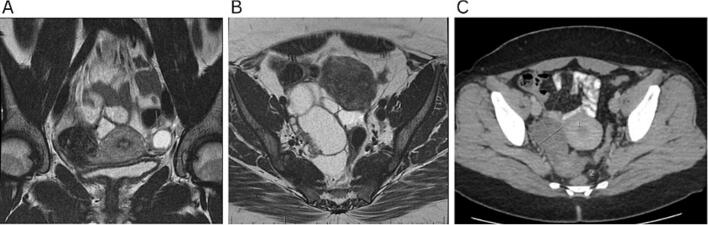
(A) Representative magnetic resonance axial image, T2-weighted, of left ovarian cyst measuring 20 mm (case 1). (B) Representative magnetic resonance axial image, T2-weighted, of right ovarian cyst measuring 96 x 50 x 56 mm (case 3). (C) Representative magnetic resonance axial image, T2-weighted, of right ovarian cyst measuring 16 mm (case 4).
